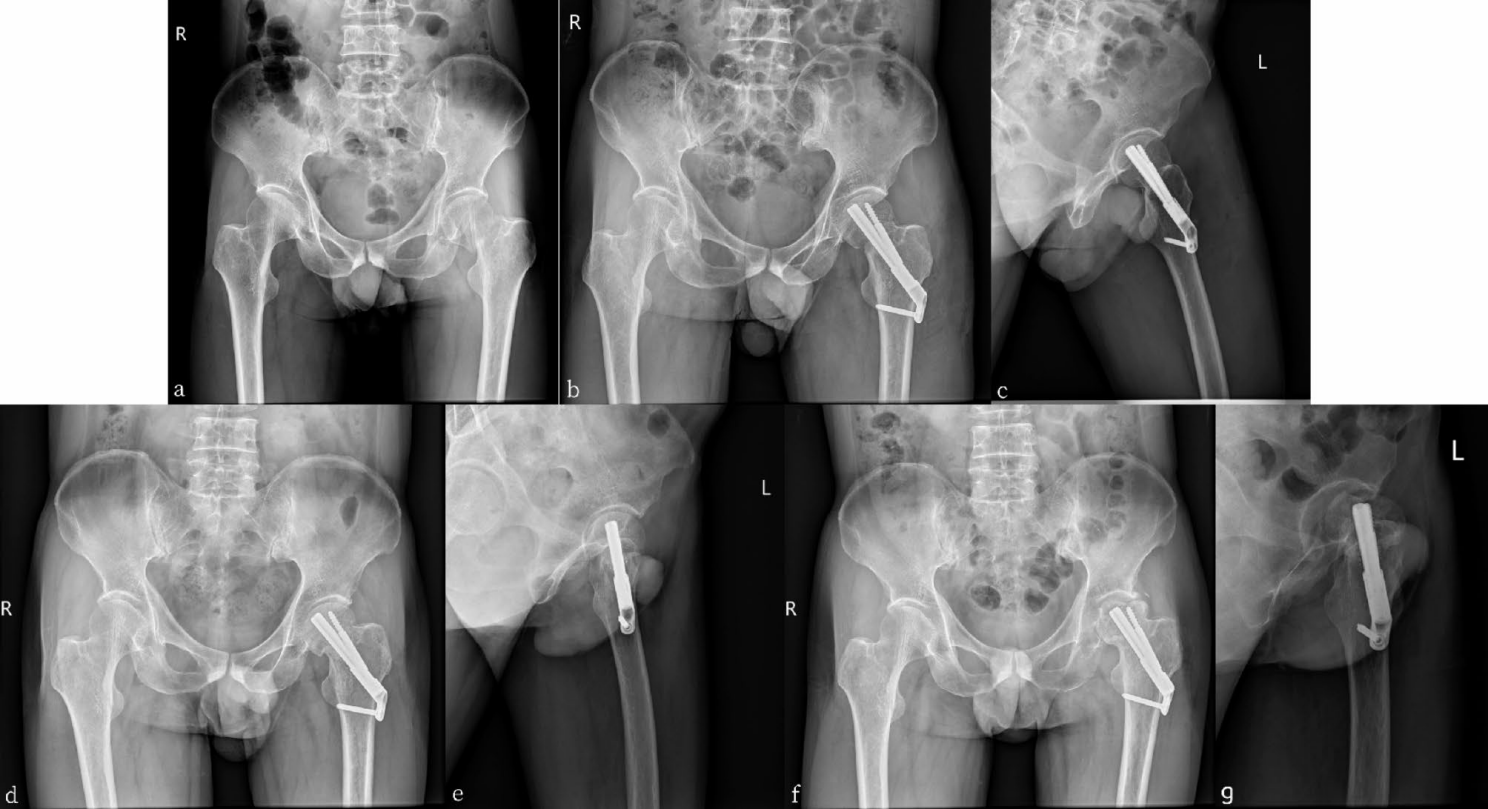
A 52-year-old male patient with left femoral neck fracture was treated with femoral neck systems(FNS). (a) Preoperative anteroposterior X-ray image. (b,c) Postoperative anteroposterior and lateral radiographs revealing satisfactory reduction of the fracture, and a satisfactory location of the FNS. (d,e) Postoperative 2-month anteroposterior and lateral radiographs showing screw sliding in the barrel of the side steel plate. Although the power rod and anti-rotation screw slid, there was no protrusion outside the side plate. (f,g) Postoperative 16-month anteroposterior and lateral radiographs showing femoral head necrosis
